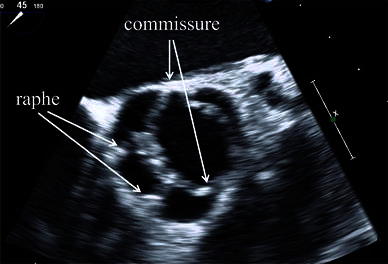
Two-dimensional transesophageal echocardiographic image of the aortic valve. Two raphes and two commissures were clearly demonstrated in systole
